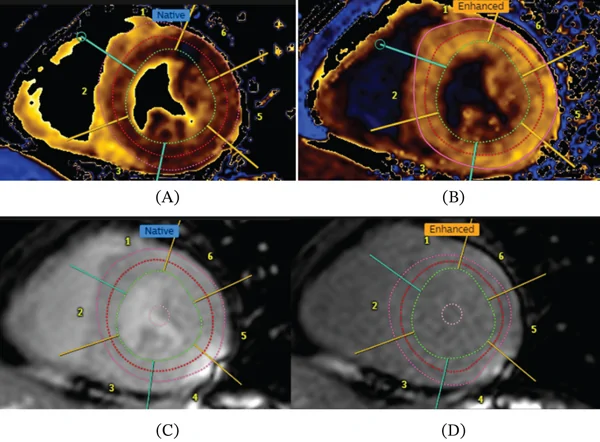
Cardiac MRI showing (A) native (noncontrast) T1 mapping, (B) enhanced (postcontrast) T1 mapping, (C) native (noncontrast) extracellular volume (ECV) mapping, and (D) enhanced (postcontrast) ECV mapping.
